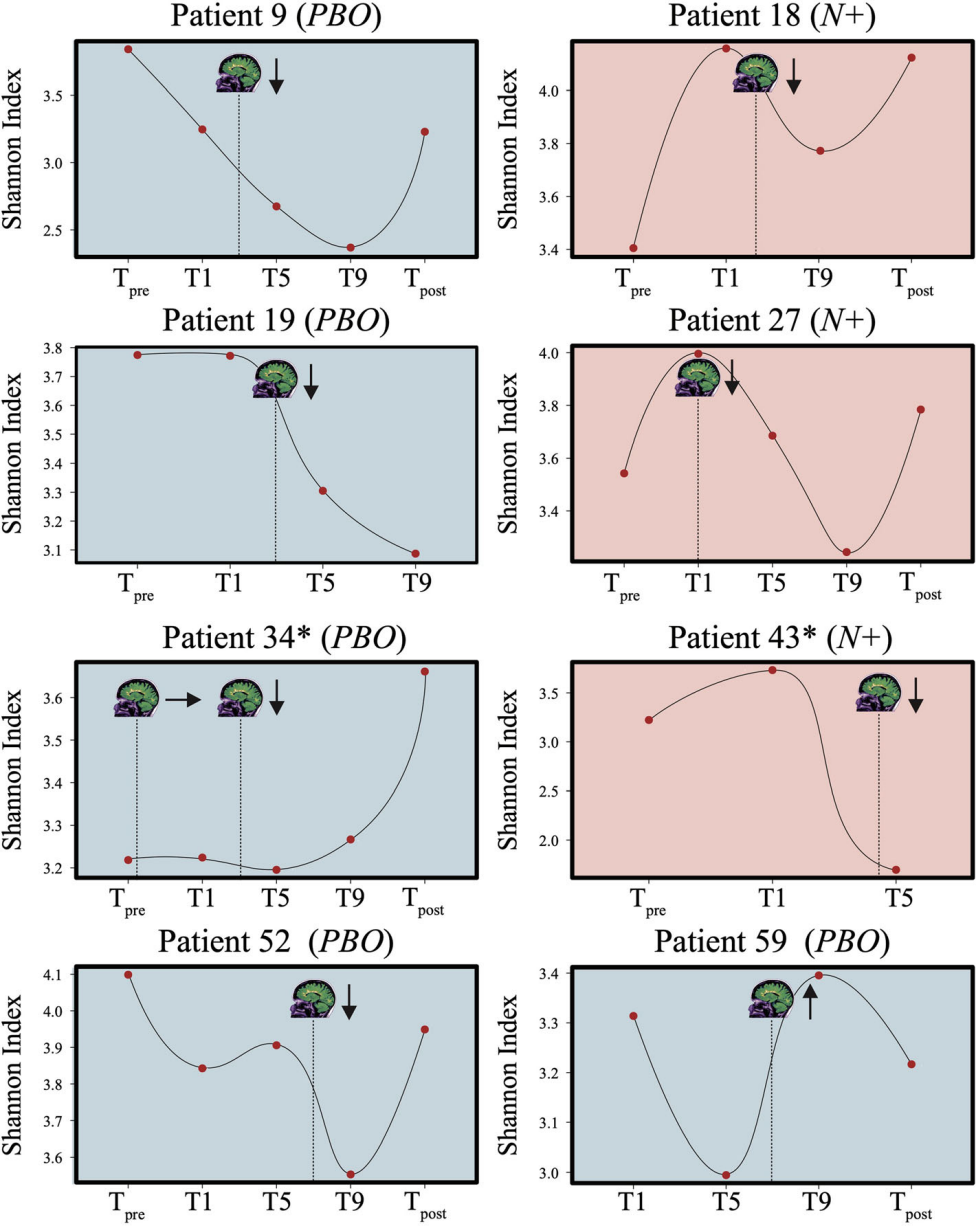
Faecal bacterial alpha diversity of volunteers with relapsing multiple sclerosis (RMS) experimentally infected with *Necator americanus* (*N*+), or placebo-treated (*PBO*). When available, trends in Shannon diversity at 1 week prior to the beginning of the study (T_pre_), at 1, 5, and 9 months post-infection/placebo treatment (T1, T5, and T9, respectively), and 2 months post-anthelmintic treatment (T_post_) are shown for both *N*+ (light red) and *PBO* (blue) subjects who suffered a clinical relapse over the course of the WIRMS trial. The stylised MRI scan indicate the timepoint(s) at which clinical relapses and new MRI lesions were recorded, whilst arrows indicate whether faecal bacterial alpha diversity increased, decreased or remained unaltered following the relapse. Asterisks (*) indicate patients who were administered steroid treatment (methylprednisolone, 2500 to 3000 mg, over 3 to 5 days) following the clinical and radiological relapse
